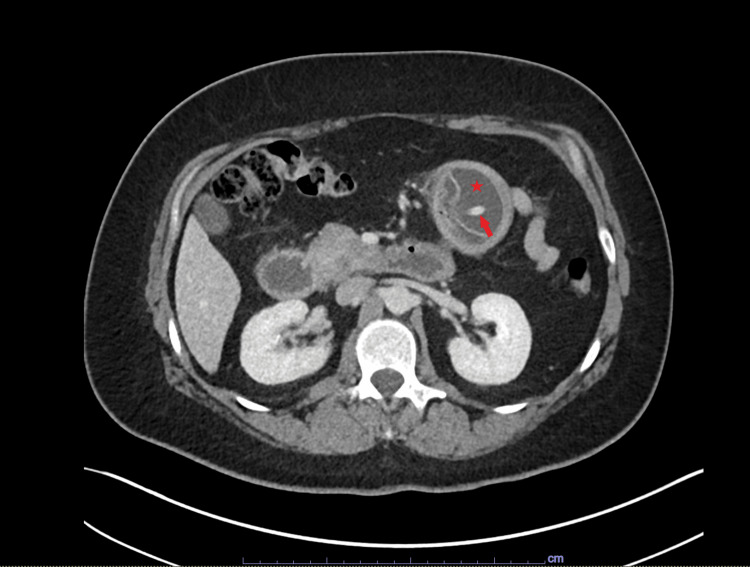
Cross-sectional computed tomography image demonstrating a device with a catheter, with a surrounding fluid-filled balloon in the proximal jejunum (arrow) The arrow indicates the catheter. The star marks the fluid-filled balloon encircling the catheter.
